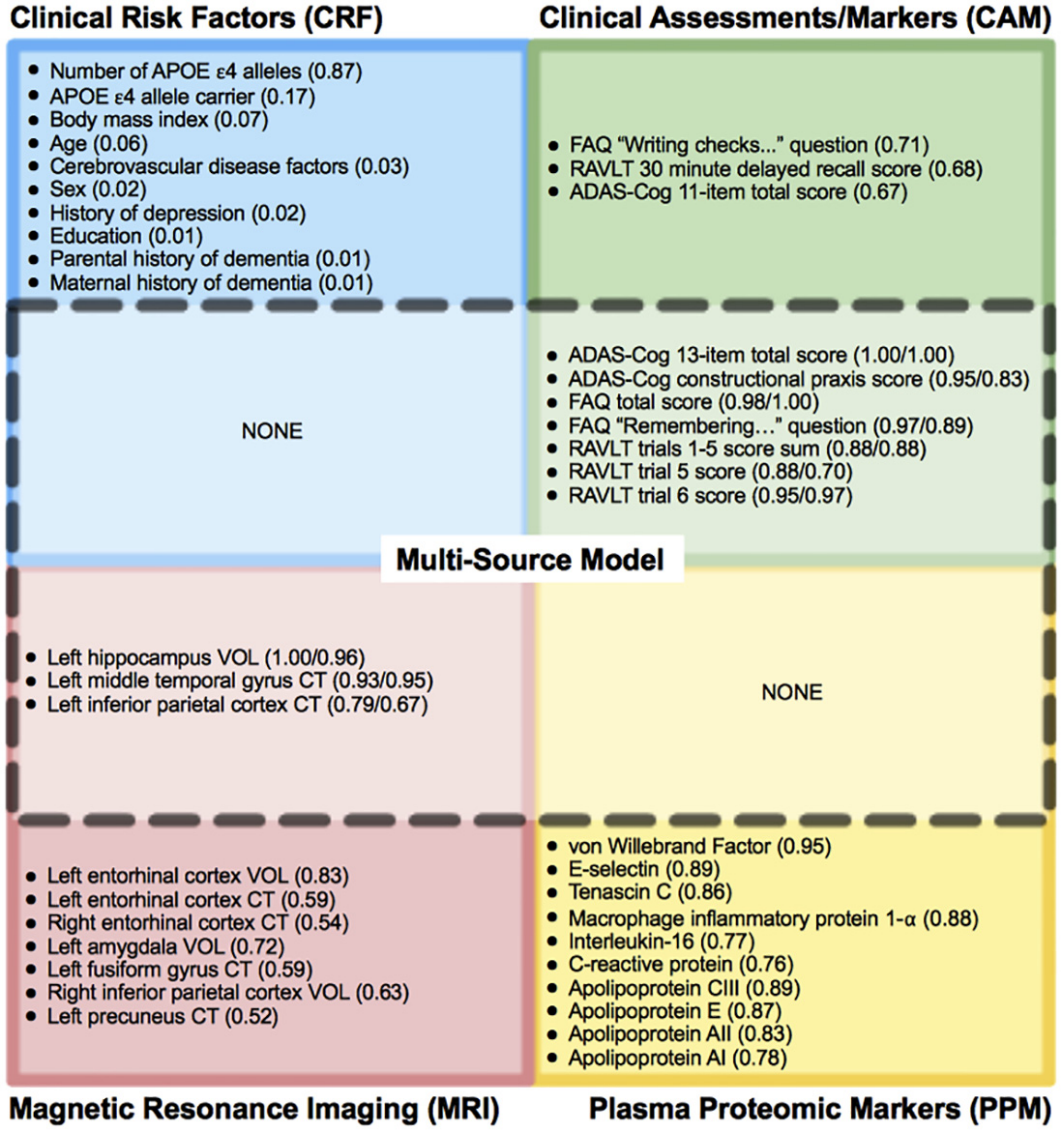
Top 10 most frequently selected features as baseline predictors of MCI-to-dementia progression. Features are shown separately for each single-source model: CRF (blue), CAM (green), MRI (red), PPM (yellow). A subset of these features (dashed line) was selected as part of both the single-kernel (CONCAT) and the multiple-kernel (MKL-Gaussian) multi-source models and included only CAM and MRI features. The selection frequency across 100 trials of the 10x10 cross-validation experiment is shown in parentheses as: (#) for single-source model only or (#/#) for both single/multi-source (MKL-Gaussian) models. APOE = apolipoprotein E, ADAS-Cog = Alzheimer's Disease Assessment Scale–Cognitive sub-scale, FAQ = Functional Activities Questionnaire, RAVLT = Rey Auditory Verbal Learning Test, VOL = volume, CT = cortical thickness.
